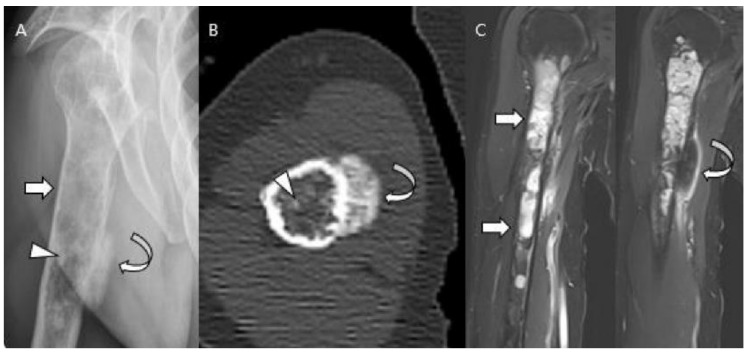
Dedifferentiated chondrosarcoma of the humerus in a 54-year-old man. (A) Plain radiograph shows an extensive mixed lytic and sclerotic lesion in the humerus with endosteal scalloping (arrow). Note the chondral-type mineralization in the intramedullary cavity (arrowhead) and the densely osteoid-type mineralization at the juxtacortical area (curved arrow). (B) Axial CT scan also reveals the intramedullary chondral-type (arrowhead) and the juxtacortical dense osteoid-type (curved arrow) mineralization. (C) Coronal T2-weighted images with fat suppression show high signal intramedullary lesion (arrows) with osteoblastic extraosseous extension (curved arrow), suggesting a dedifferentiated component of osteosarcoma.
